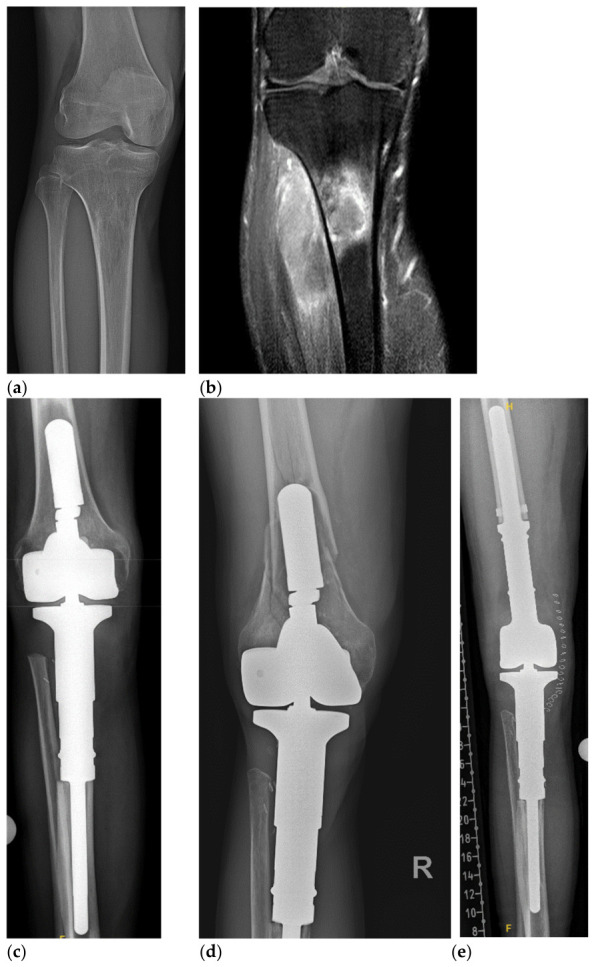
48 year old patient with a Ewing sarcoma of the proximal tibia: (a) radiography of the proximal tibia at initial diagnosis, (b) MRI of the proximal tibia at initial diagnosis, (c) radiography after tumor resection and reconstruction with a proximal tibia replacement, (d) radiography of the knee joint with periprosthetic fracture of the distal femur, and (e) radiography after conversion to a total knee replacement.
